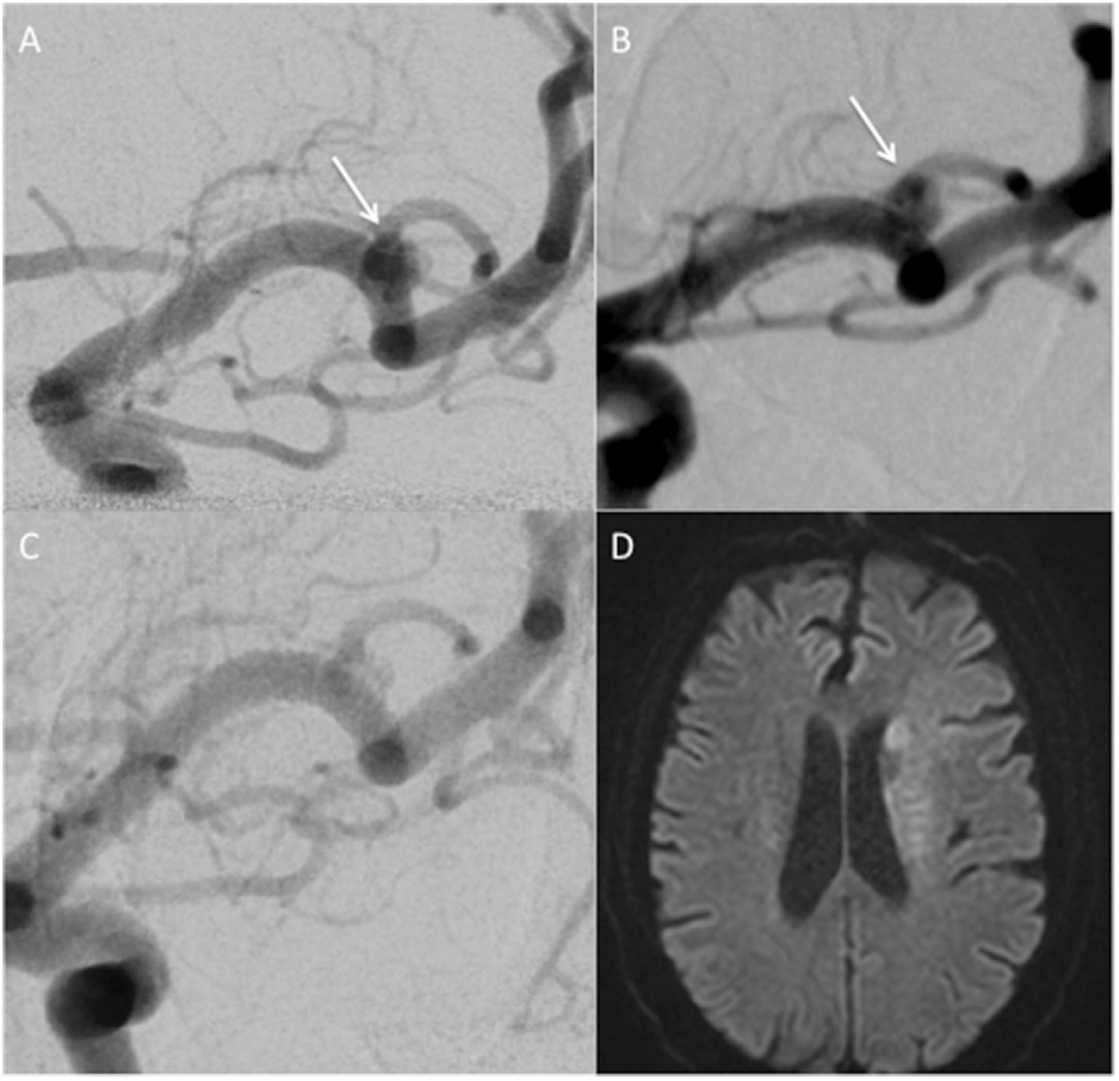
A patient treated with a single p64 FDS for a middle cerebral artery bifurcation anerurysm. An incidental untreated bifurcation aneurysm (A). The same aneurysm in a different projection shows a wide necked saccular MCA bifurcation (B). There were no intra-operative complications and immediately post-procedure the patient awoke with baseline neurology. Five days post-procedure, the patient developed a right hemiparesis. Catheter angiography at this time (C) showed all branches were patent with anterograde flow and minimal filling of the aneurysm. An MRI showed restricted diffusion (D).
